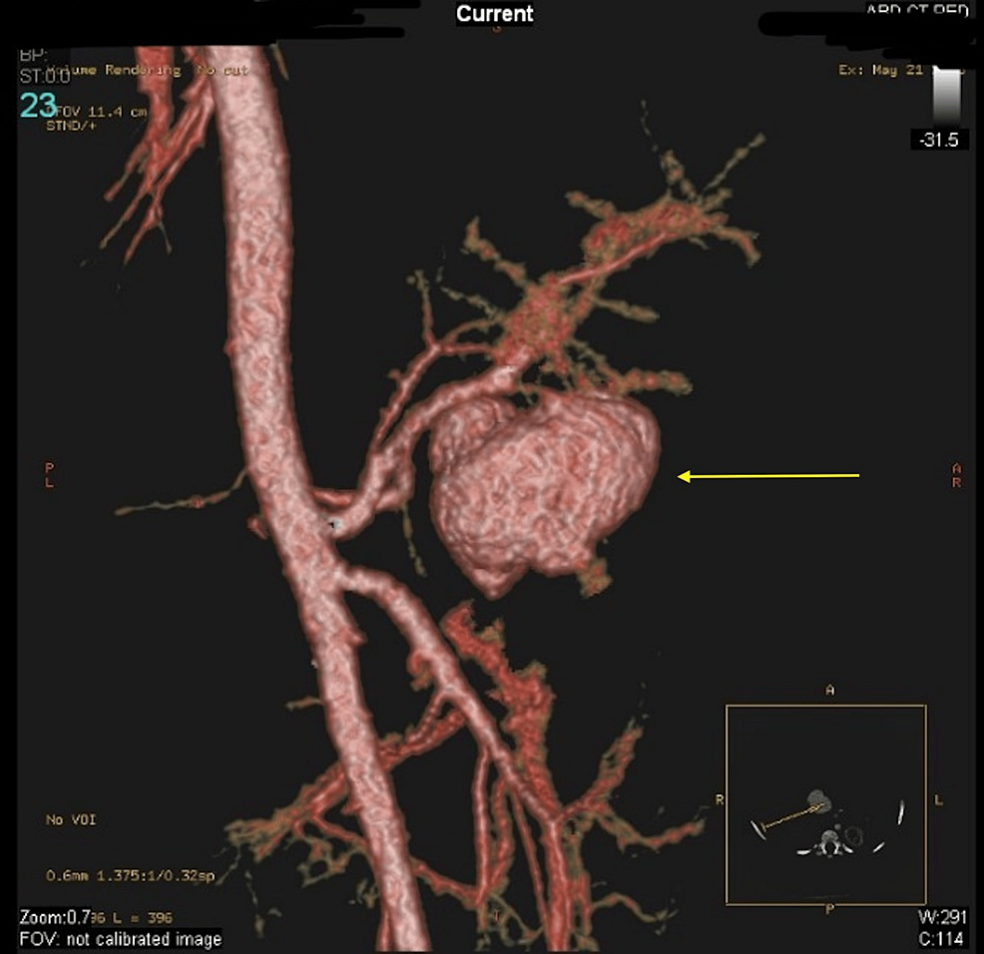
3D CT images confirming the communication of the pseudoaneurysm from the hepatic artery with a turbulent flow at this level (arrow) 3D CT: three-dimensional computed tomography
